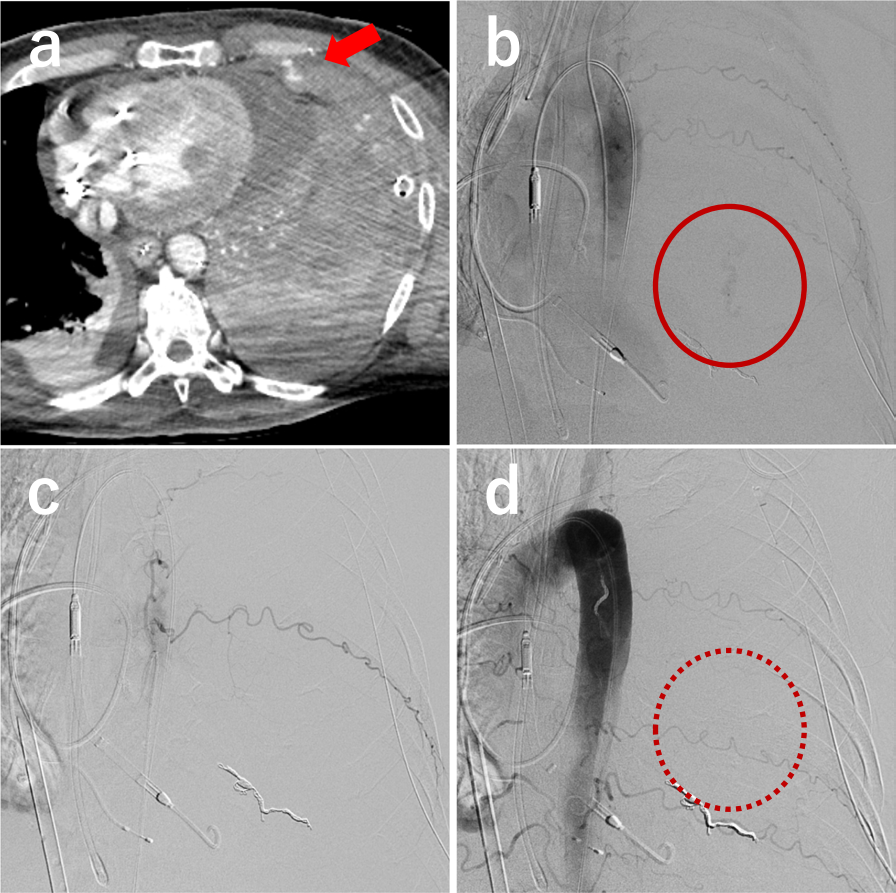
CECT and TAE on the 12th day of the patient’s hospitalization. a Arterial phase of a thoracic CECT showing a left hemothorax and residual contrast media EV into the left thoracic cavity (arrow). b Aortography with a pigtail catheter advanced from the brachial artery to the descending aorta showing an EV from the 3rd left posterior intercostal artery (circle). c Approaching from the ECMO return cannula, the left intercostal artery was targeted and embolized using a gelatin sponge. d Final aortography confirming the disappearance of the EV (dotted circle). CECT: chest contrast-enhanced computed tomography, TAE: transarterial embolization, EV: extravasation, ECMO: extracorporeal membrane oxygenation
